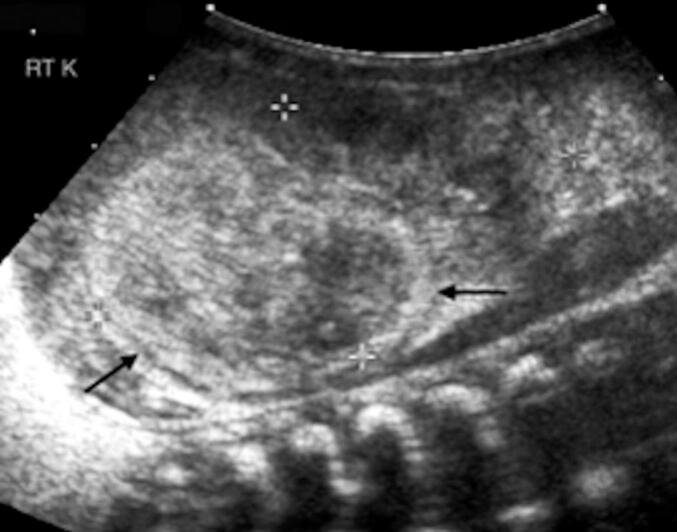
“Vascular ring” sign on ultrasound.
